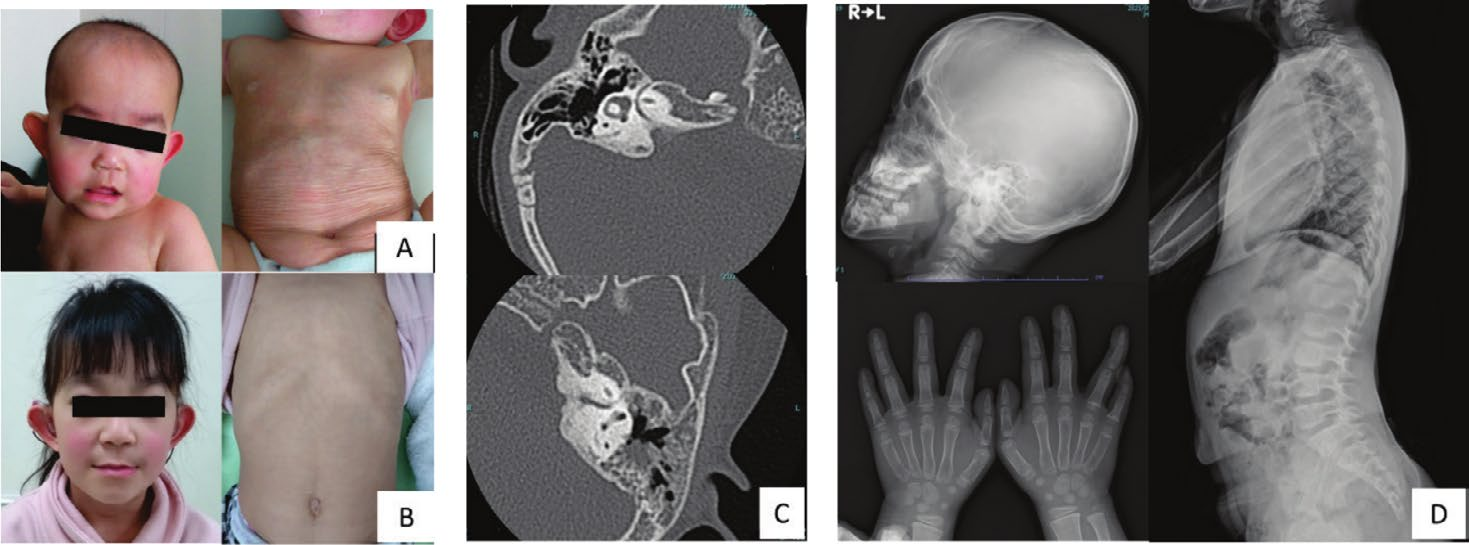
Clinical photographs and radiological imaging of facial, skin, and bone features. (A) 10‐month‐old (B) 6‐year‐old (C) Inner ear hypoplasia is shown by a temporal bone CT scan at 2 years old. (D) Short middle phalanges of hands, no signs of bone sclerosis in the whole body.
